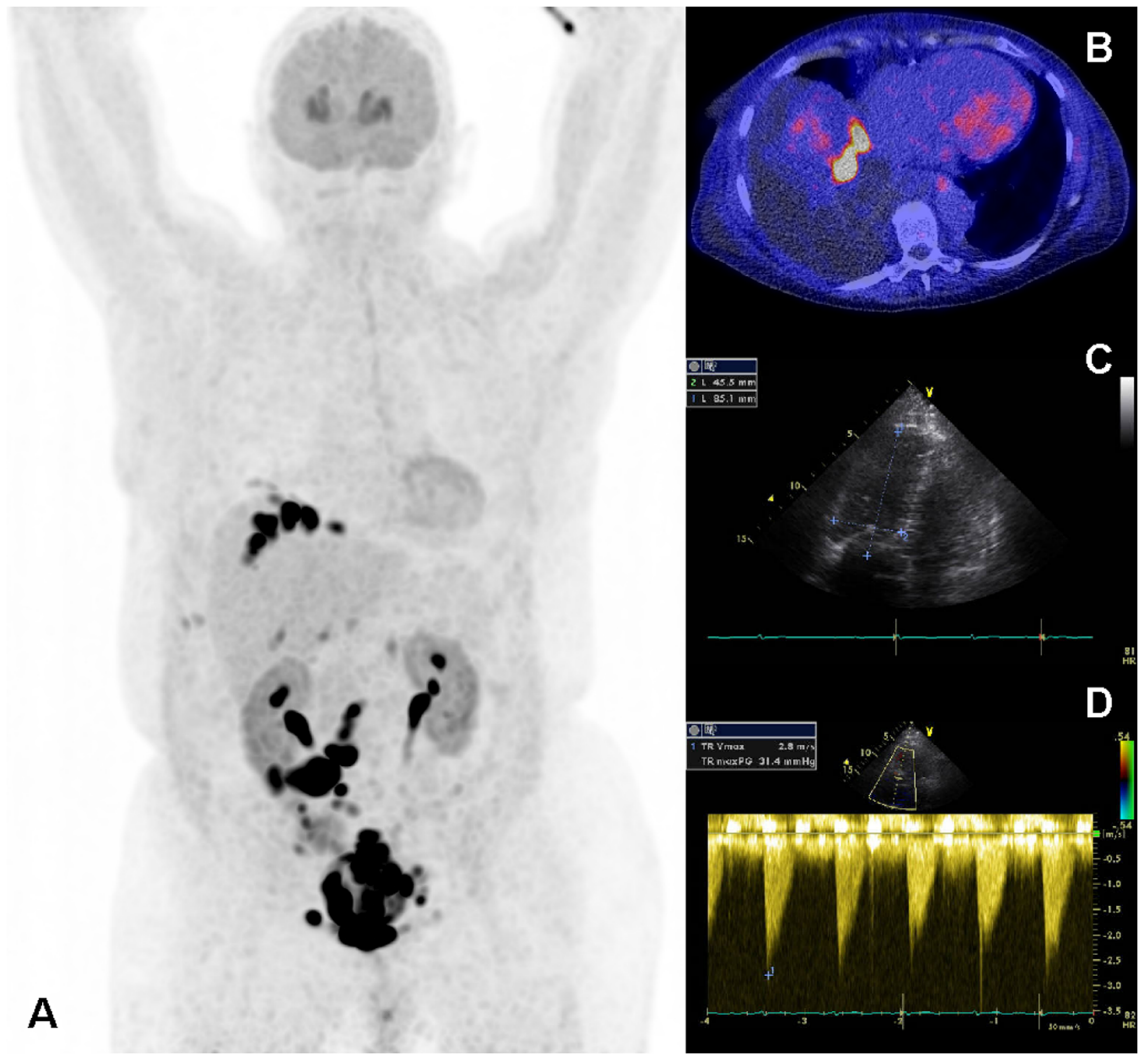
Maximum intensity projection (A) of an ^18^F-FDOPA PET/CT scan in a patient with echocardiographic signs of carcinoid heart disease, but without myocardial metastases on the ^18^F-FDOPA PET/CT scan. B: Fused PET/CT image showing only physiological tracer distribution in the myocardium. The intense uptake in liver is due to liver metastases. Ascites surrounding the right liver half. C: Measurements of the right ventricle (85 mm long, 46 mm wide). D: Continuous wave Doppler of the tricuspid valve (peak gradient 31 mmHg), indicating tricuspid insufficiency.
